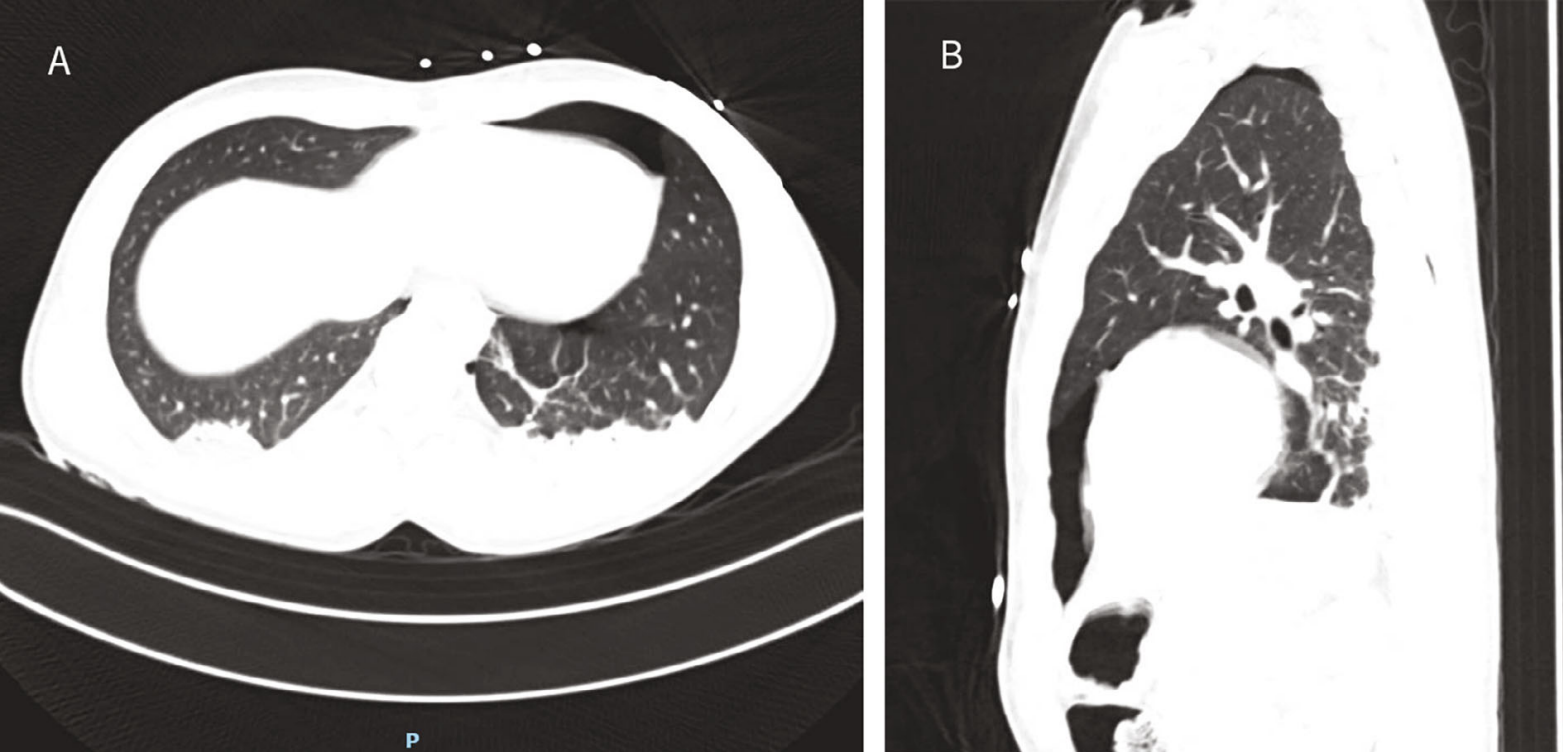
Thoracic CT scan made in the emergency department, showing a small ventral left‐sided pneumothorax. (A) Axial view. (B) Sagittal view.
